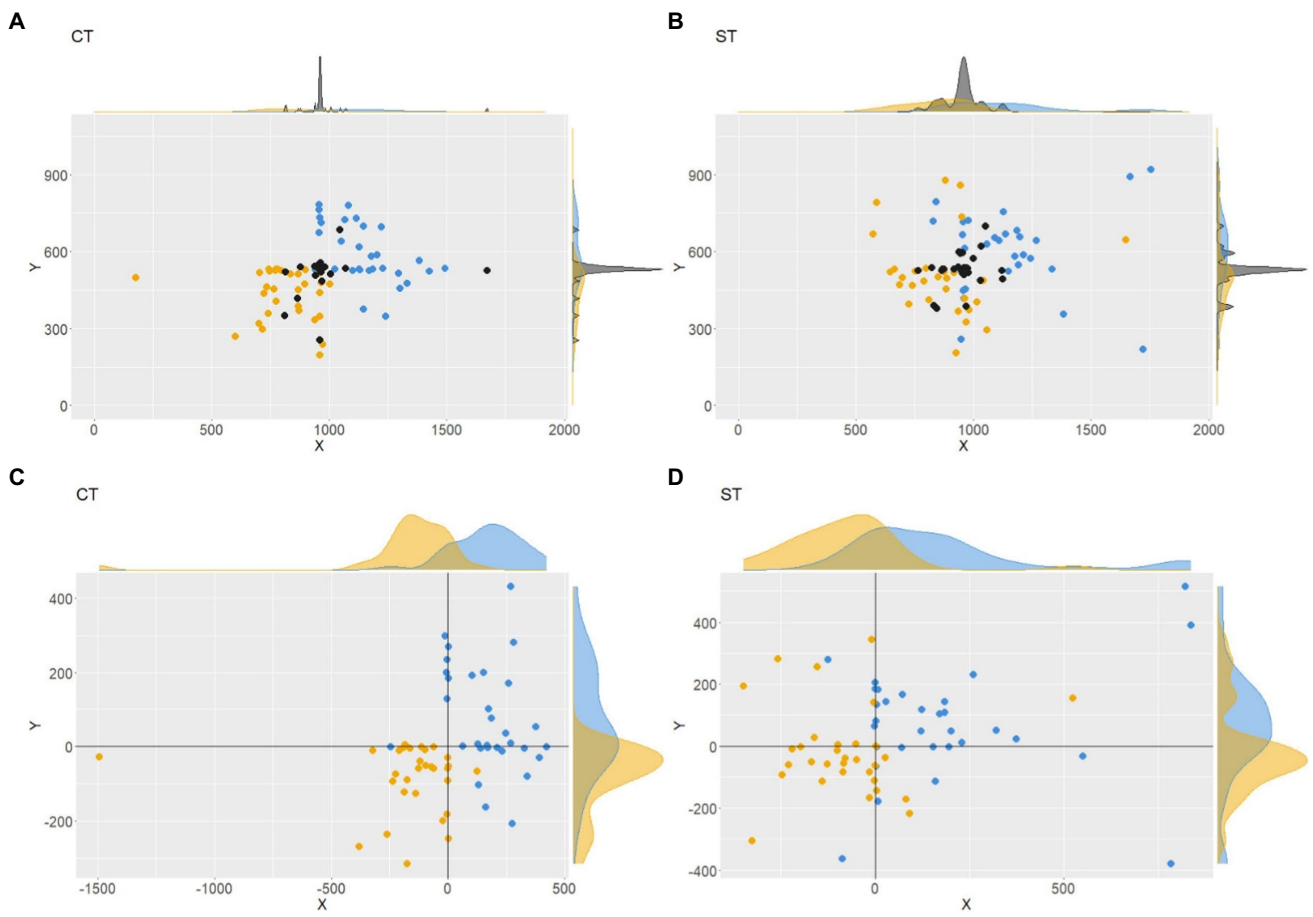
Absolute positions of circles in CT (A) and ST (B). Each yellow, black, and blue dot expresses the past, present, or future position of a participant. The same results are plotted as positions relative to the present circle/sphere of the same participant for CT (C) and ST (D).
